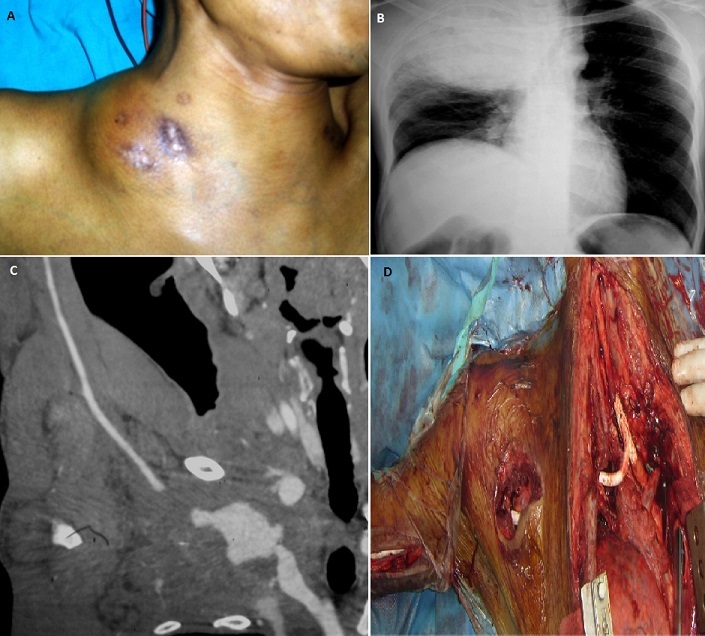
A) a supraclavicular mass, with central necrotic lesions; B) X-ray showing an opacity of the right hemithorax with left tracheal deviation; C) computed tomographic angiography showing a false aneurysm of the right subclavian artery; D) bypass graft from brachio-cephalic trunk to the humeral artery and carotid artery
